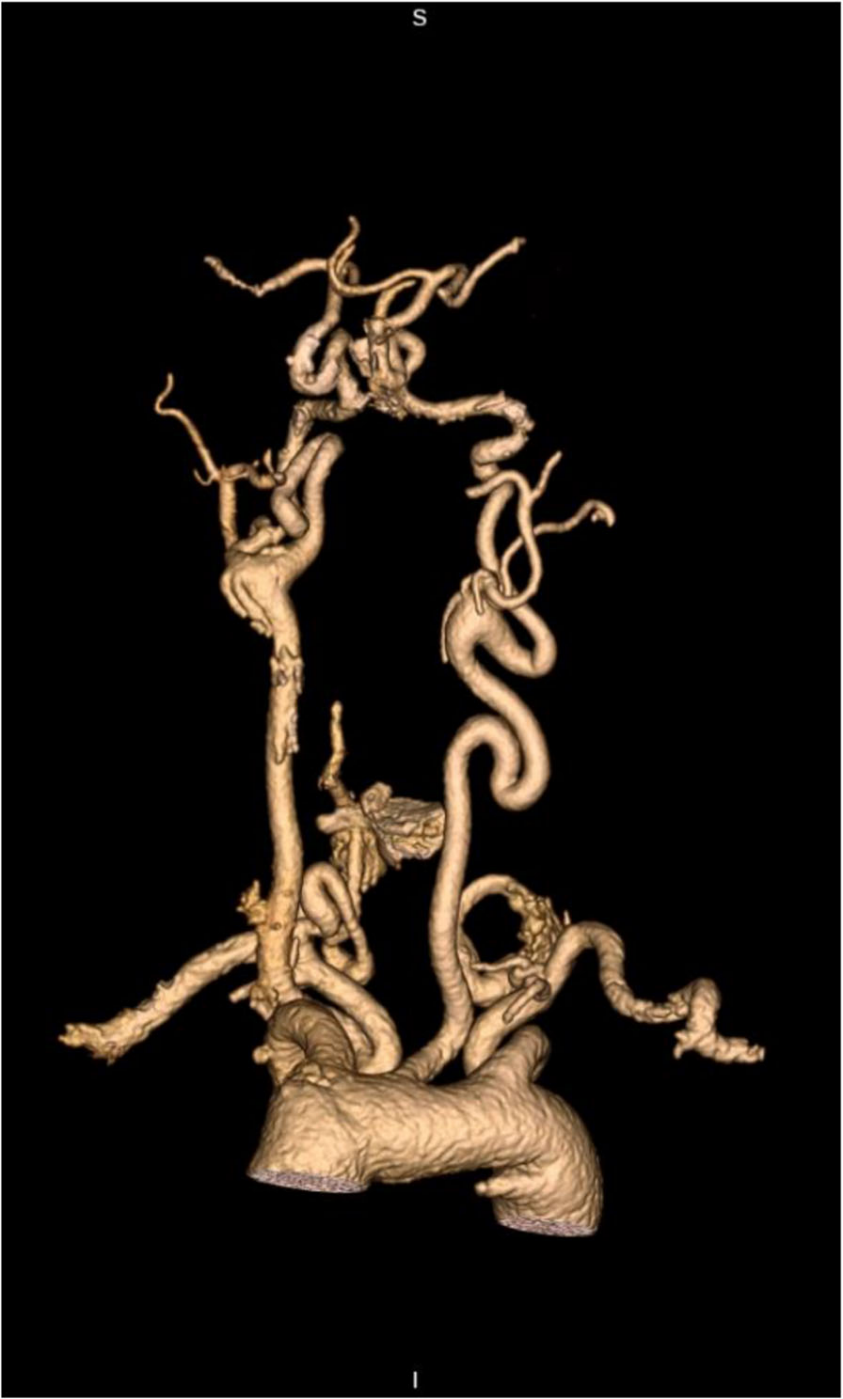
MRI angiogram of the patient’s vertebral, carotid and subclavian arteries at the age of 19, demonstrating severe arterial tortuosity. Permission to publish the image was given from the Department of Radiology and Nuclear Medicine at Oslo University Hospital
